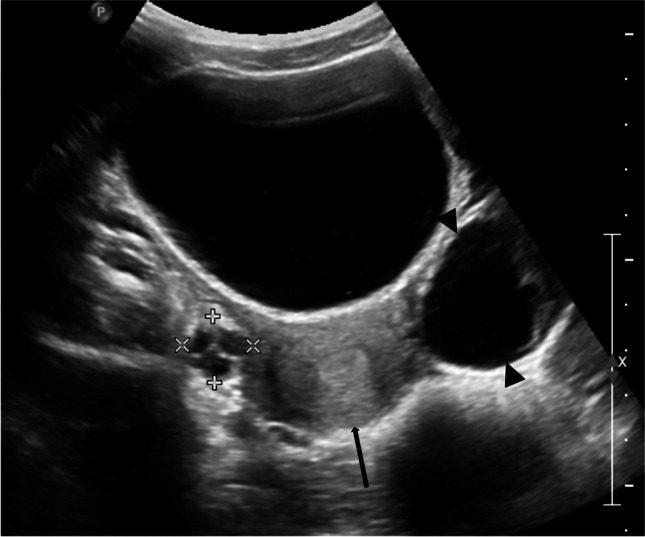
Axial gray-scale pelvic ultrasound image in a 5-year-old girl with McCune-Albright syndrome shows a large follicular cyst on the left ovary (*arrowheads*) and a stimulated uterus (*arrow*). The normal right ovary is marked by cursors
